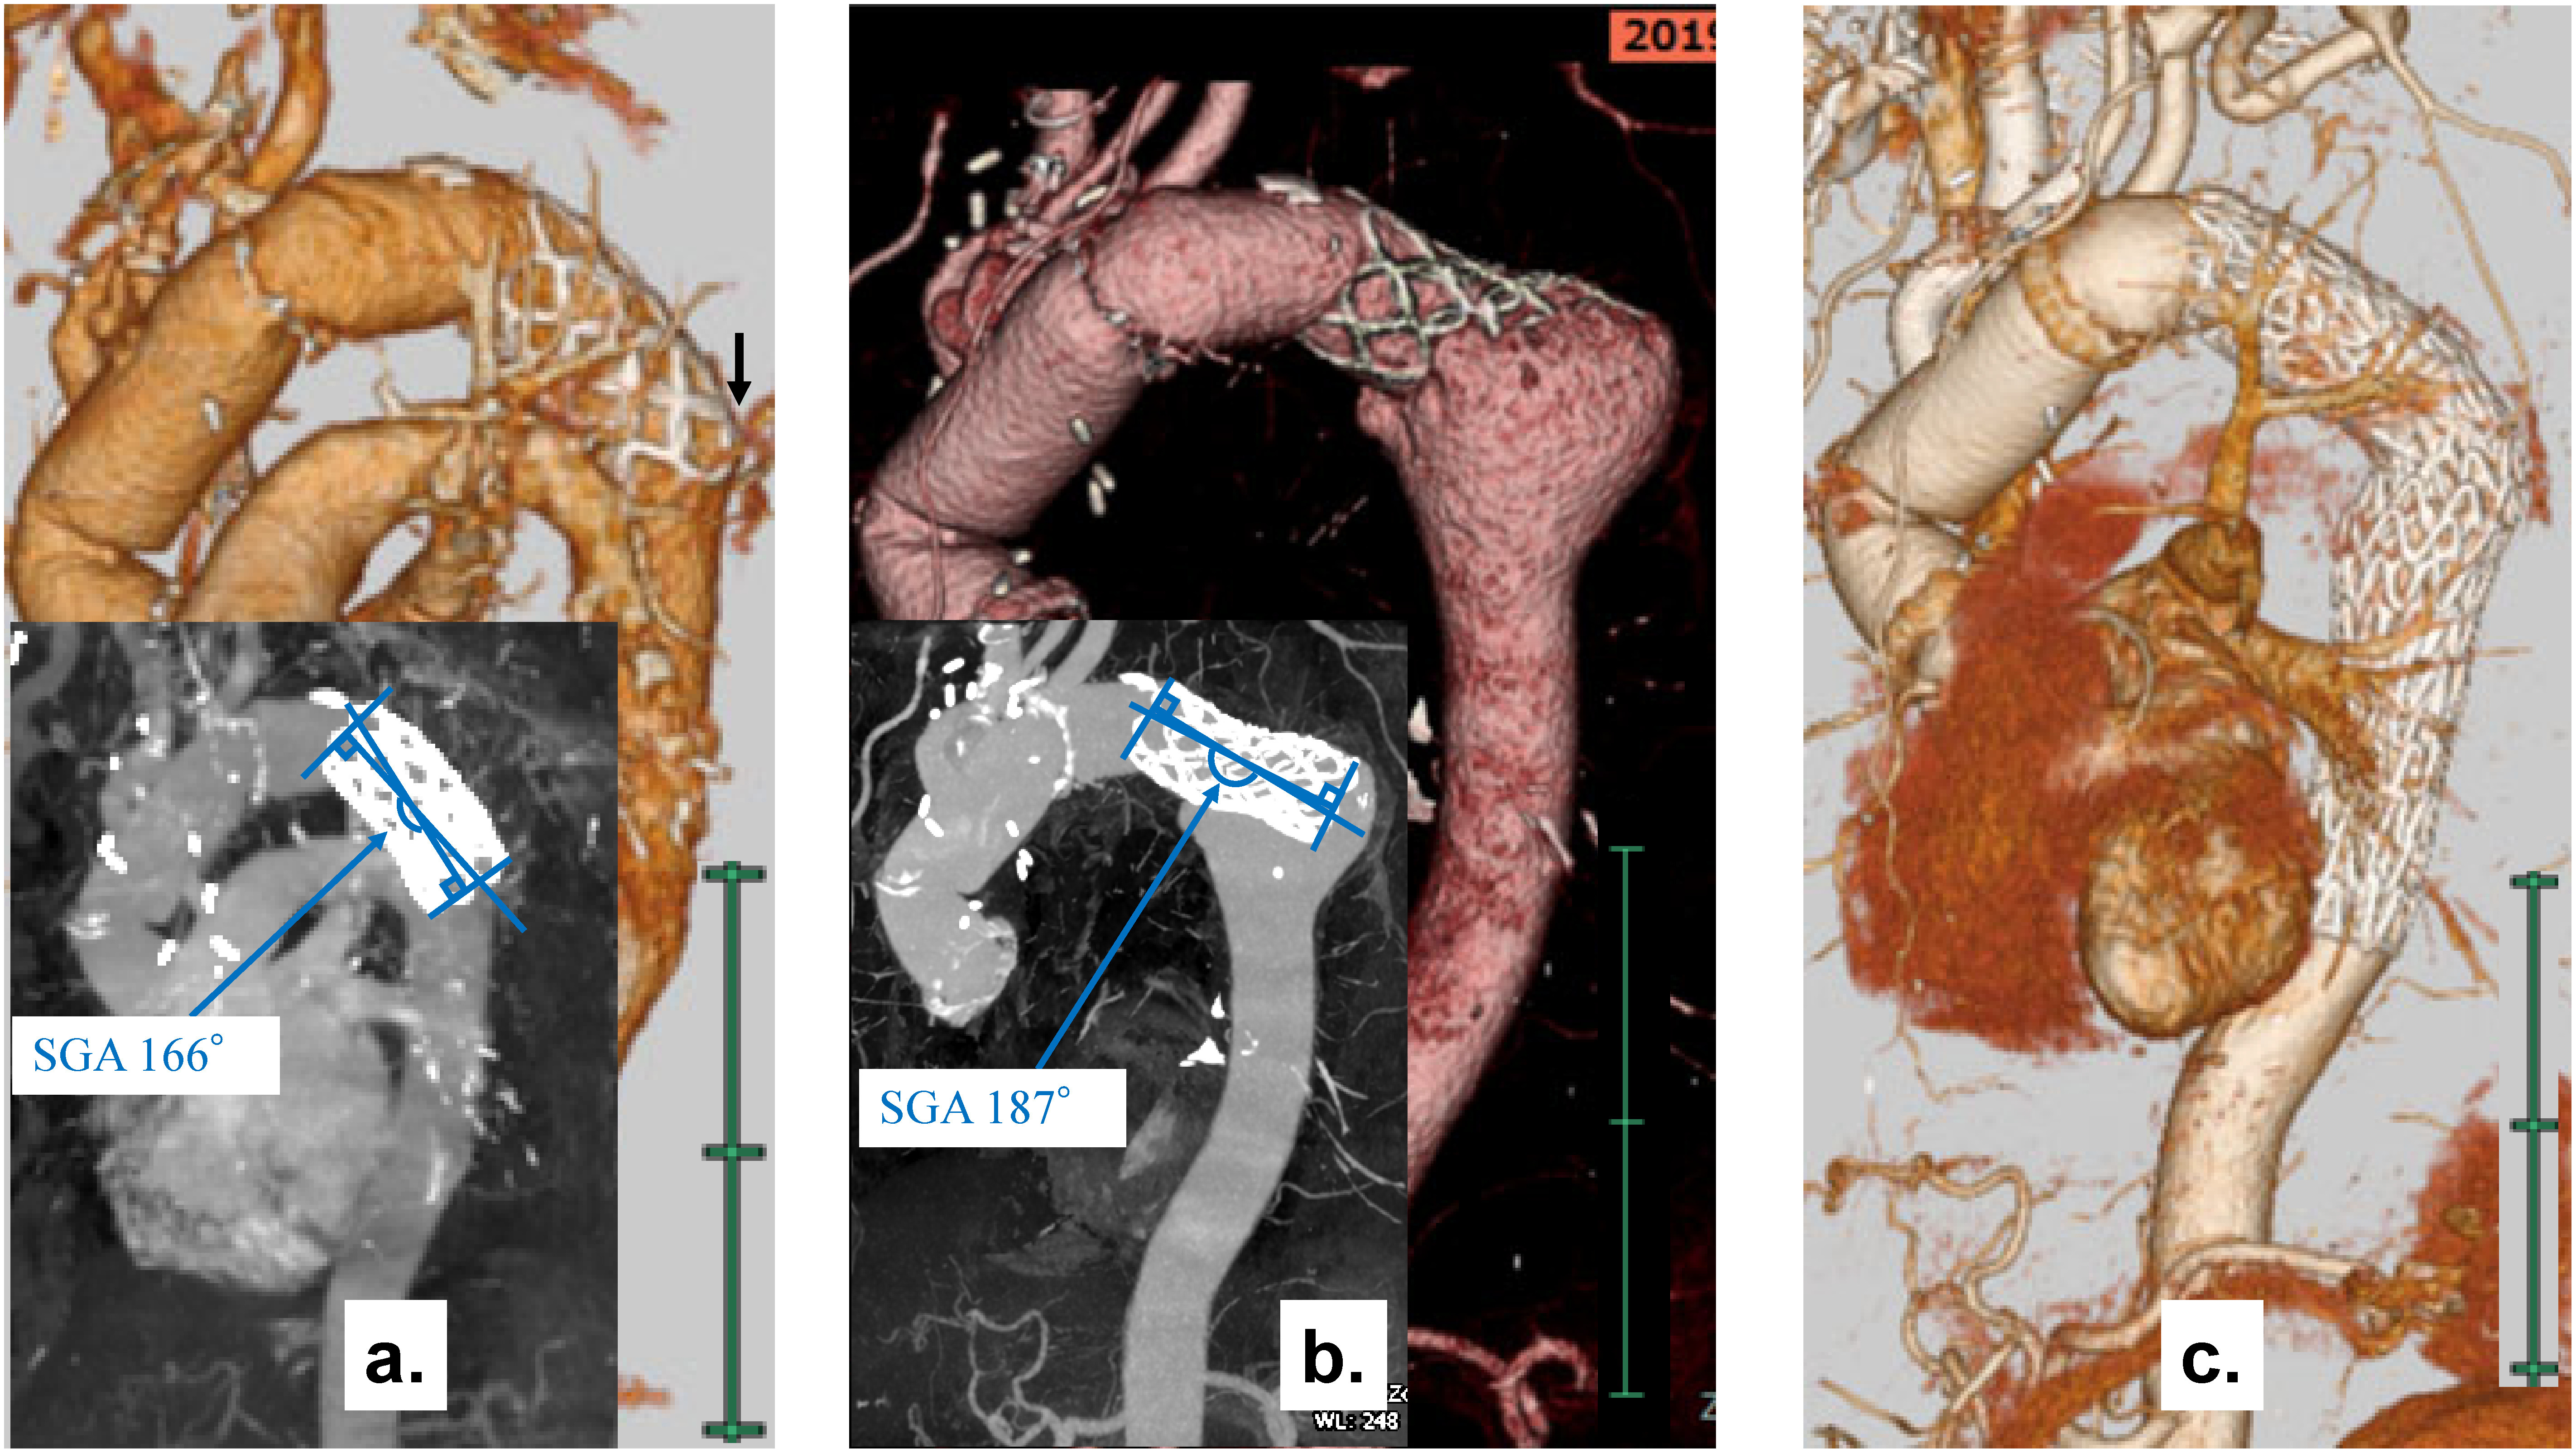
Fig. 2 Follow-up CT images with three-dimensional reconstruction after the total arch replacement with the frozen elephant trunk procedure. (a) Five months after the surgery. A small DSINE (arrow) appeared in the distal end of the JOSG at the greater curvature of the descending aorta. The SGA was slightly increased to 166°. (b) Seven months after the surgery. Rapid progression of DSINE and aneurysm in the descending aorta were shown, and the stent graft was straightened to the SGA of 187°. (c) Post-procedural CT showed that the DSINE disappeared after thoracic endovascular aortic repair.
